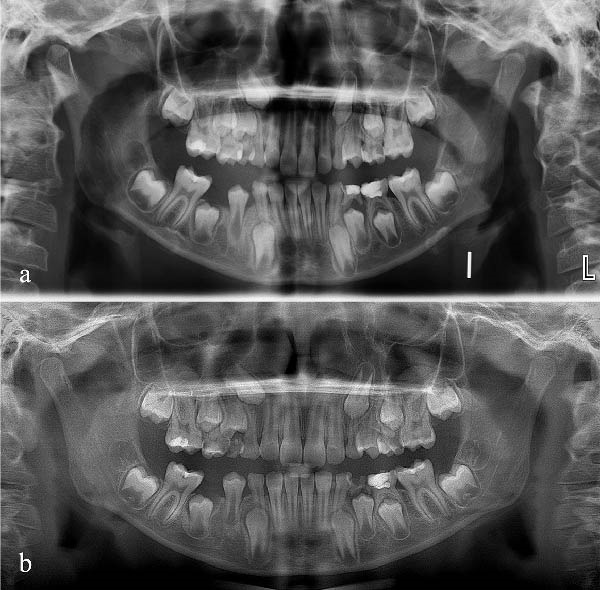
(a) Radiographic resolution observed at 12‐month follow‐up. (b) Complete healing and final radiograph at 18‐month follow‐up.
